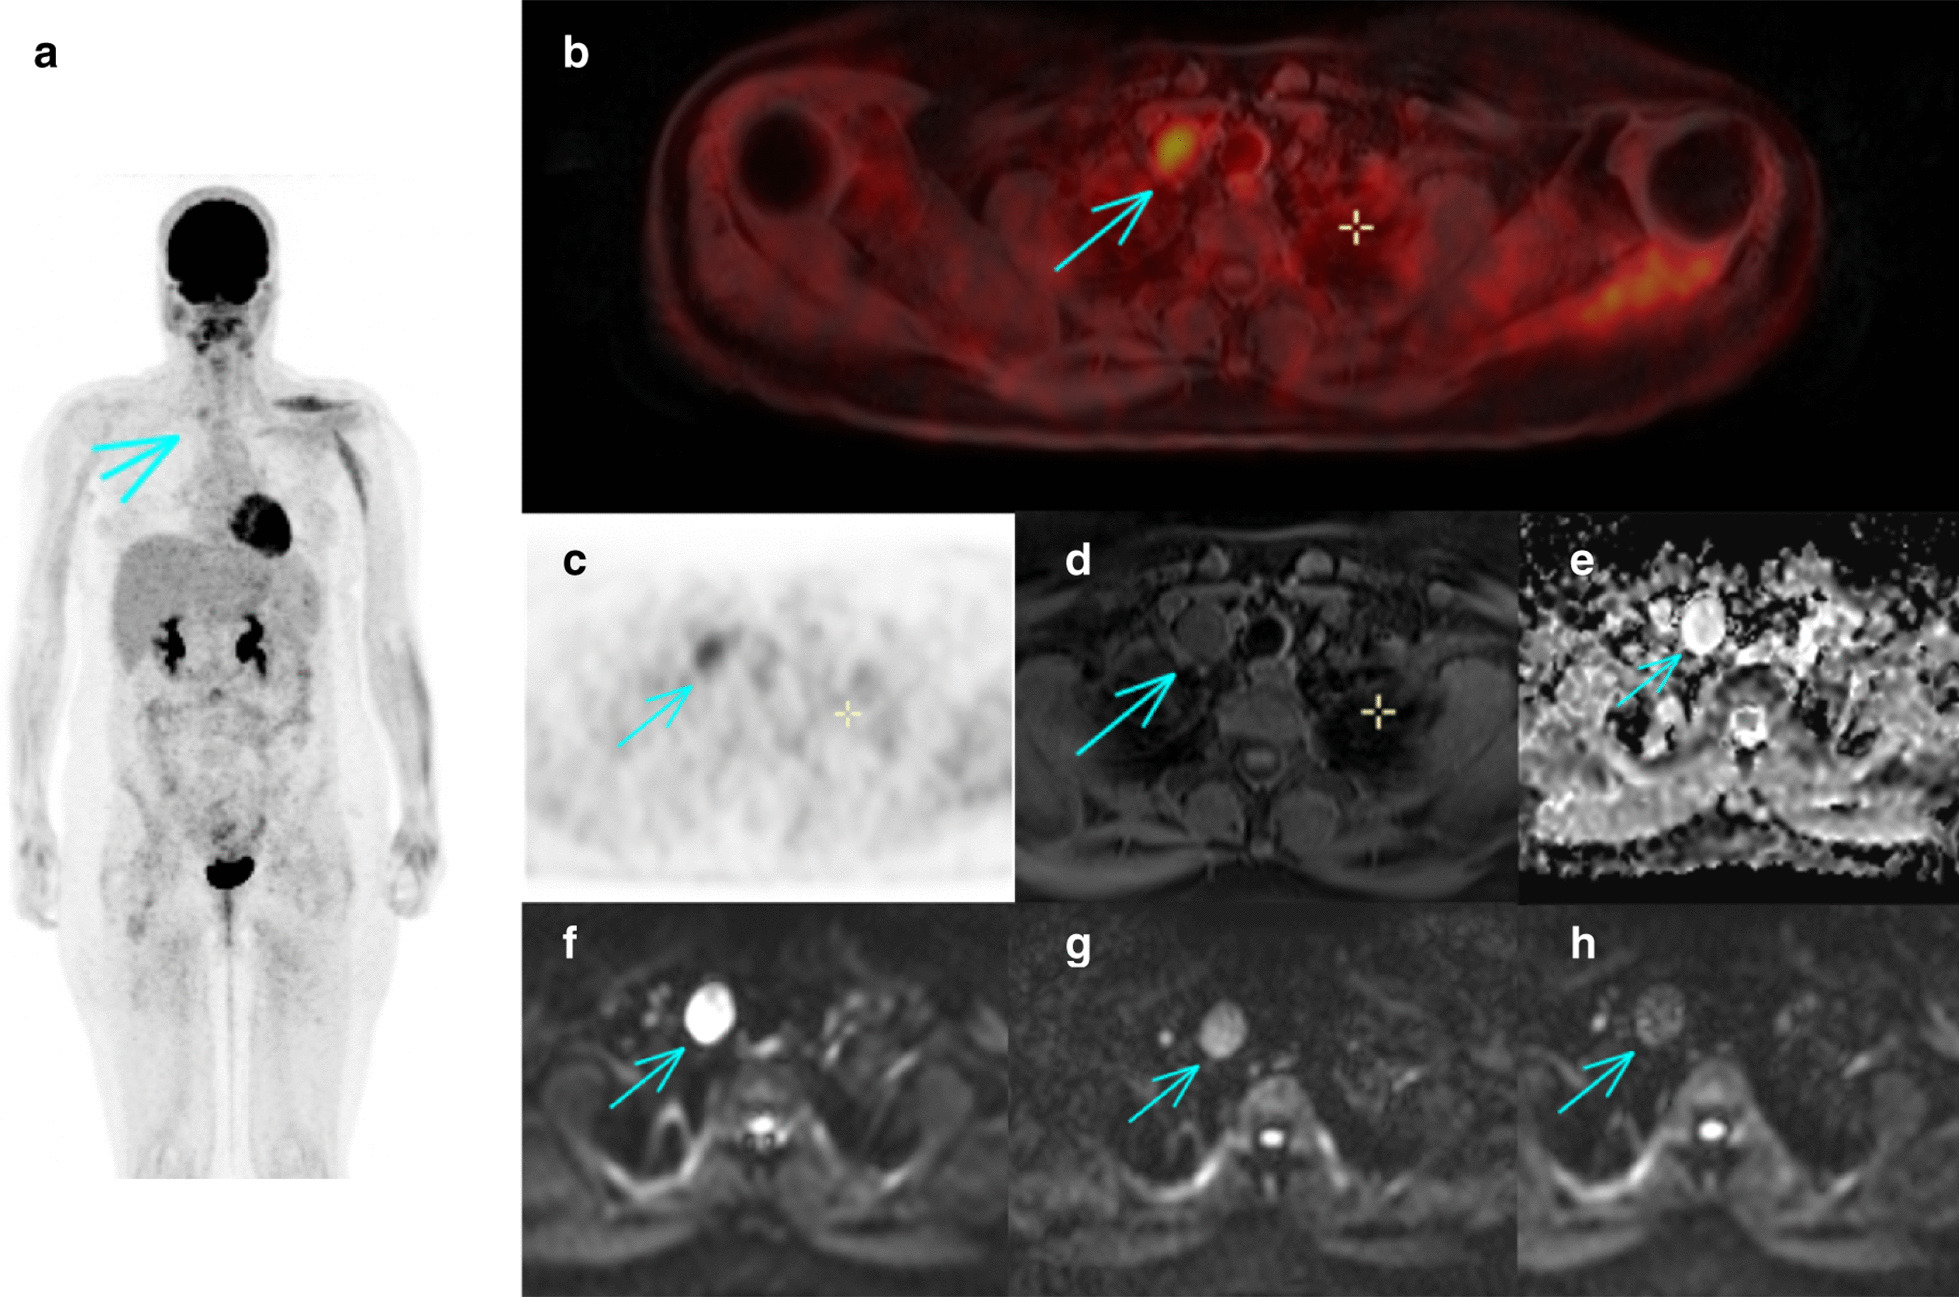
Example of a schwannoma detected by both MRI and ^[18F]^FDG-PET. From top left to down right: a Coronal maximum intensity projection (MIP) ^[18F]^FDG-PET image, b axial fused TEP/T1w image, c axial ^[18F]^FDG-PET image, d axial T1-weighted (T1w) fat-suppressed image, e axial apparent diffusion coefficient (ADC) image and f–h axial diffusion-weighted images with 3 b values (50, 400 and 800 s/mm^2^). The arrows indicate the same schwannoma in the different images
